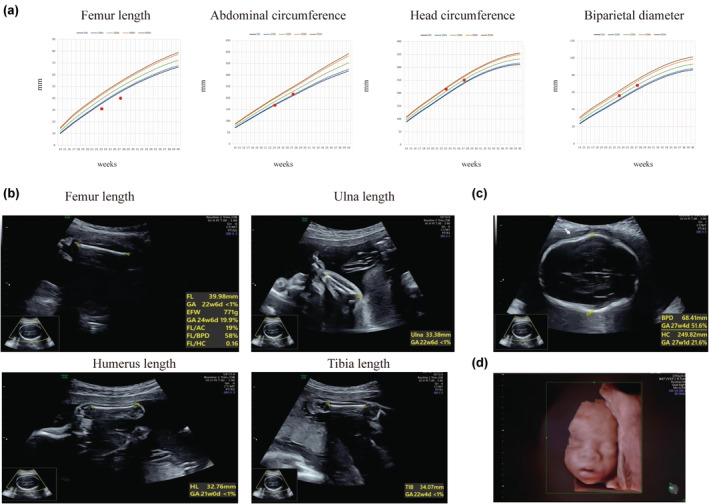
Growth parameters of the affected fetus. (a) The femur length, abdominal circumference, head circumference, and biparietal diameter of the fetus at 23 + 3 and 27 + 2 weeks. (b) Ultrasound image of the fetus at 27 + 2 weeks showing decreased humerus, ulnar, and tibial length. (c) Unilateral temporal bone depression arrow. (d) Facial features of the fetus.
